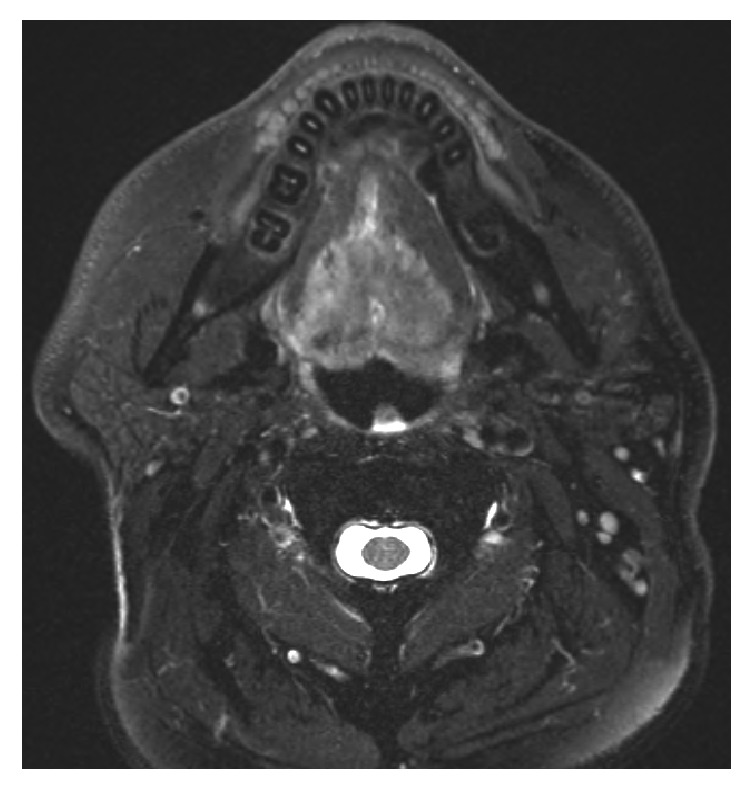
MRI of lesion in base of tongue.
